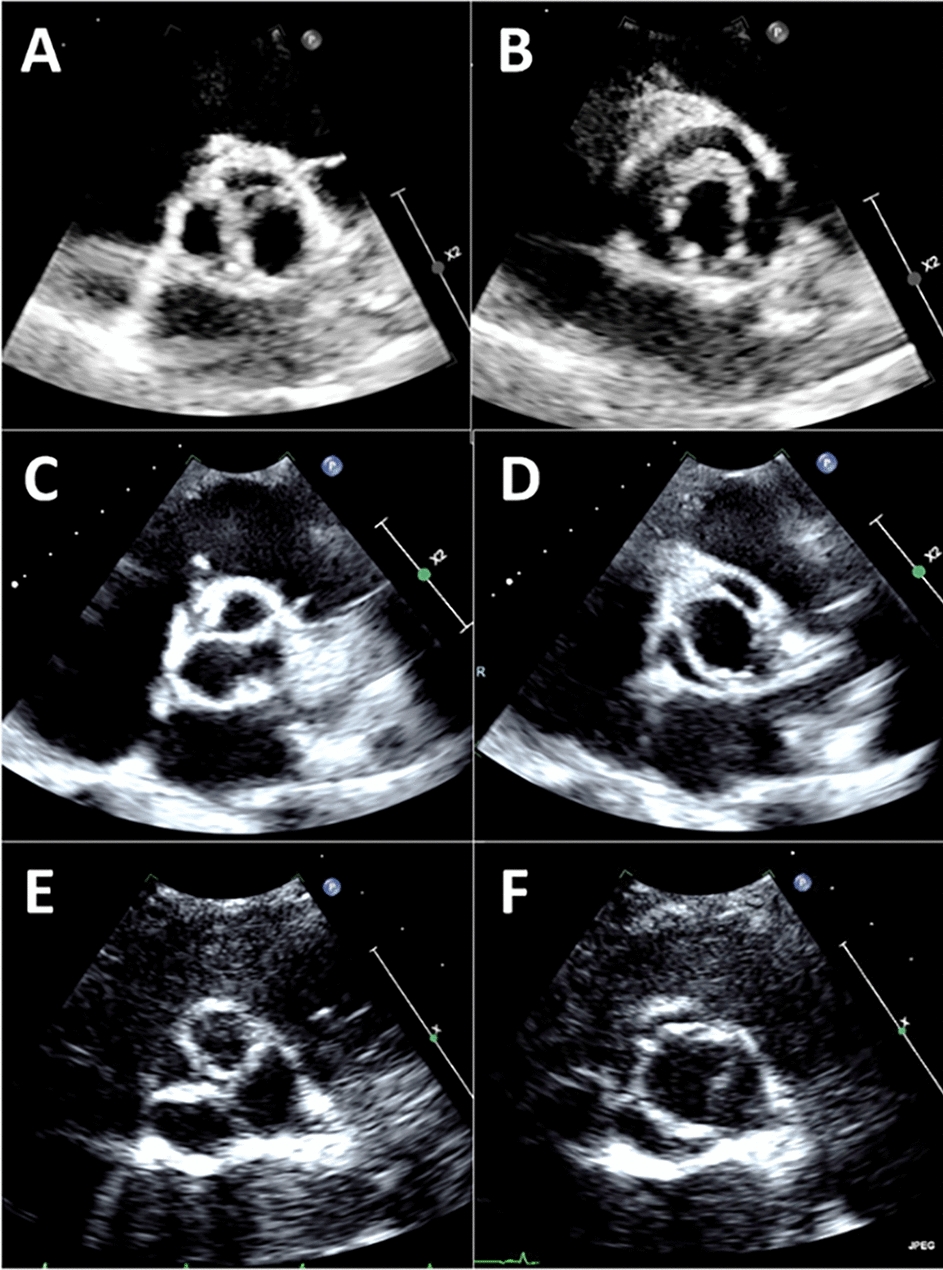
Variant anatomy of the aortic valve (AV); From a parasternal short axis, images (A) and (B) show a unicuspid AV in diastole and systole; Images (C) and (D) show a bicuspid AV with a left–right orientation and a raphe at approximately 3 o’clock in diastole and systole; Images (E) and (F) show a quadricuspid AV in diastole and systole
